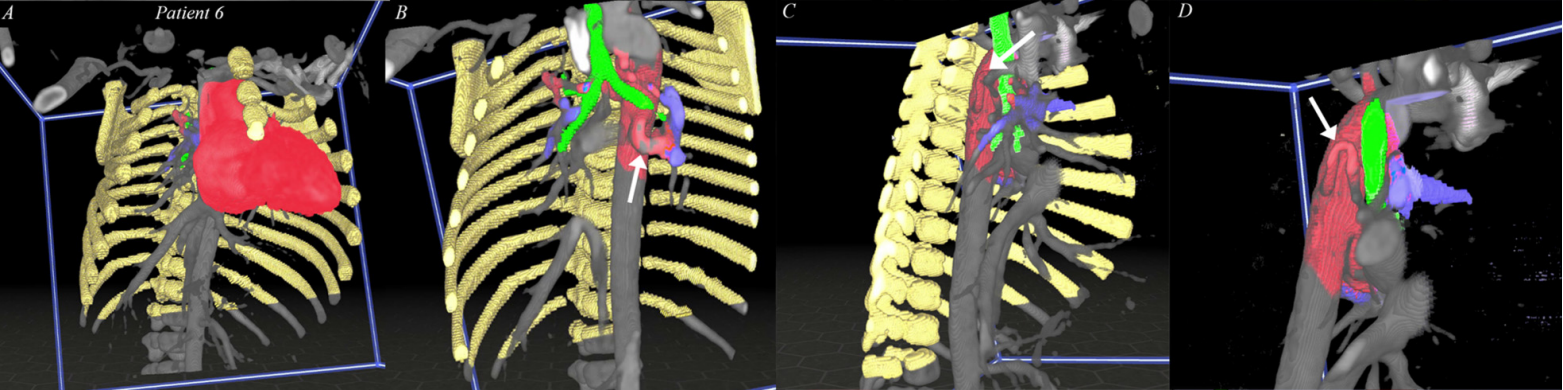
Realtime 3D-VR visualization of segmented CT scan*. (A)
